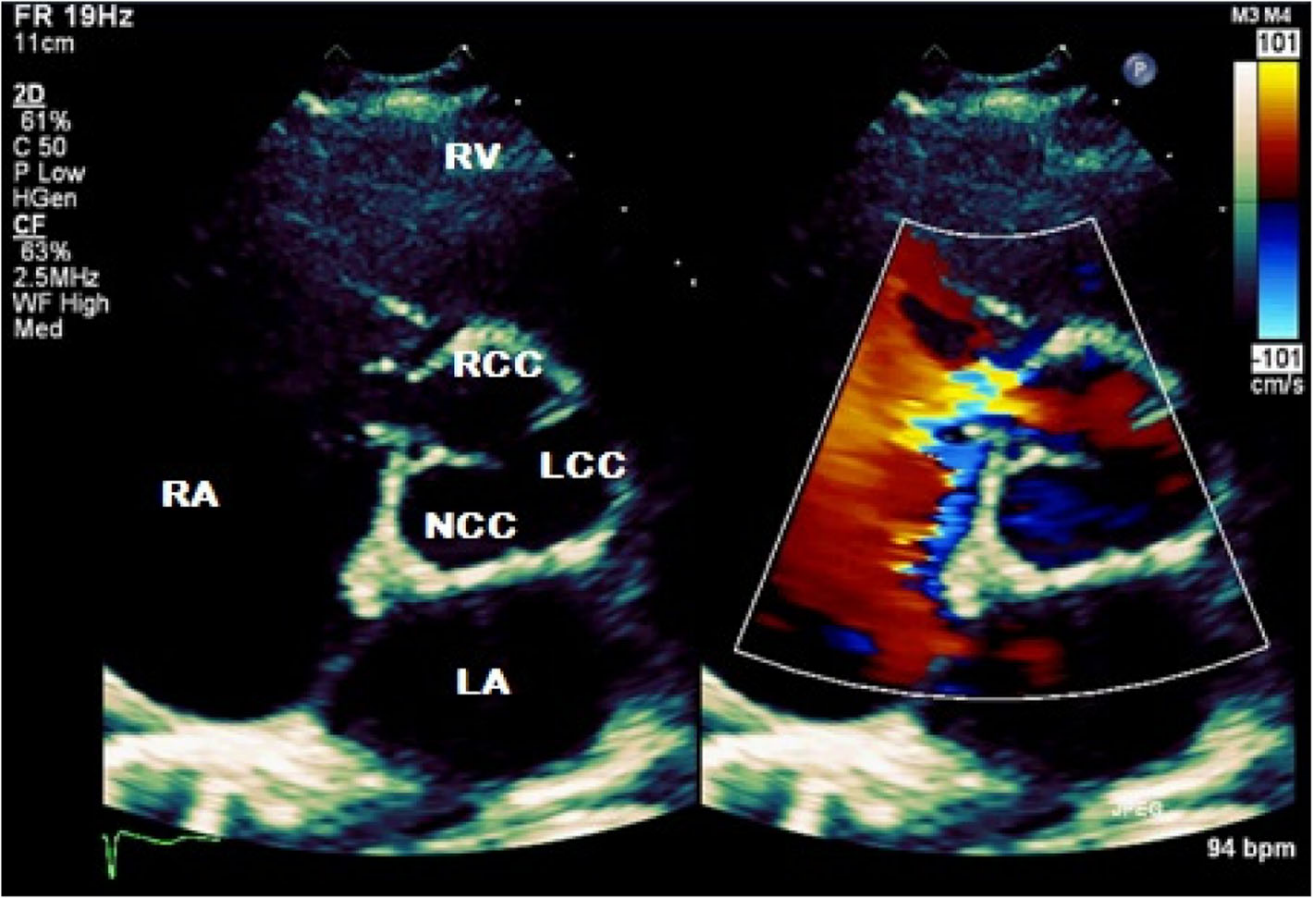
Two-dimensional transthoracic echocardiography in parasternal short axis with colour compare view demonstrating a shunt from the right coronary sinus to the right atrium. RV, right ventricle; RA, right atrium; LA, left atrium; RCC, right coronary cusp; NCC, non-coronary cusp; LCC, left coronary cusp
